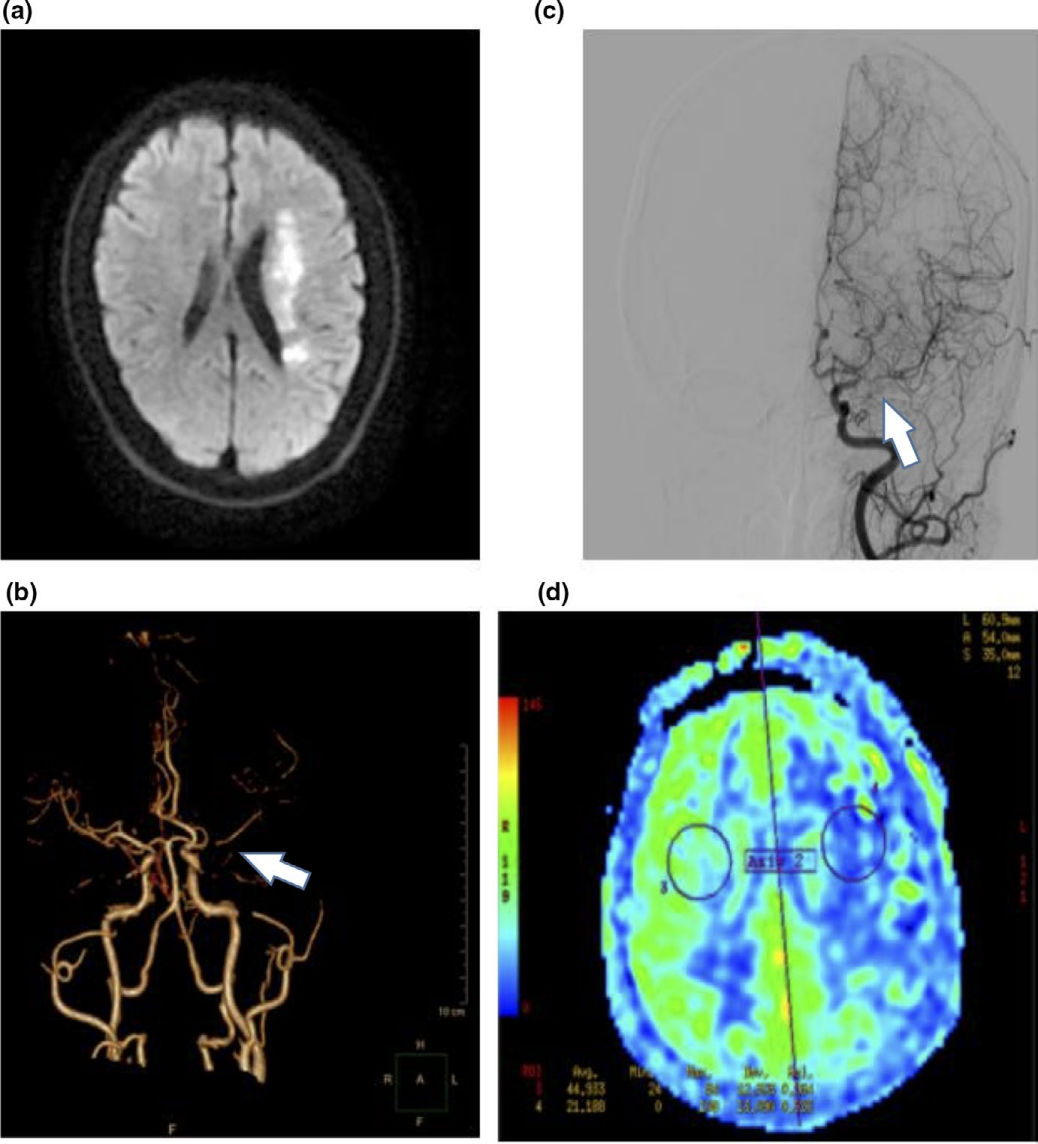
Hypoperfusion stroke mechanism in ischemic stroke patients with ICAS. Images from a 57‐year‐old man with acute cerebral infarction in the left cerebral hemisphere. When the patient was admitted to the hospital, the consciousness was clear, presented with mixed aphasiathe, the right side of the nasolabial fold became shallow, right limb muscle strength was level 0, and the left limb muscle strength was level 5. NIHSS on admission was 19 and mRS at discharge was 4. (a) DWI shows hemodynamic impairment mechanism leading to acute ischemic stroke in left hemisphere. (b,c) CTA and DSA show severe stenosis in left middle cerebral artery. The arrows indicate stenosis location. (d) Arterial spin‐labeling shows hypoperfusion in left hemisphere
